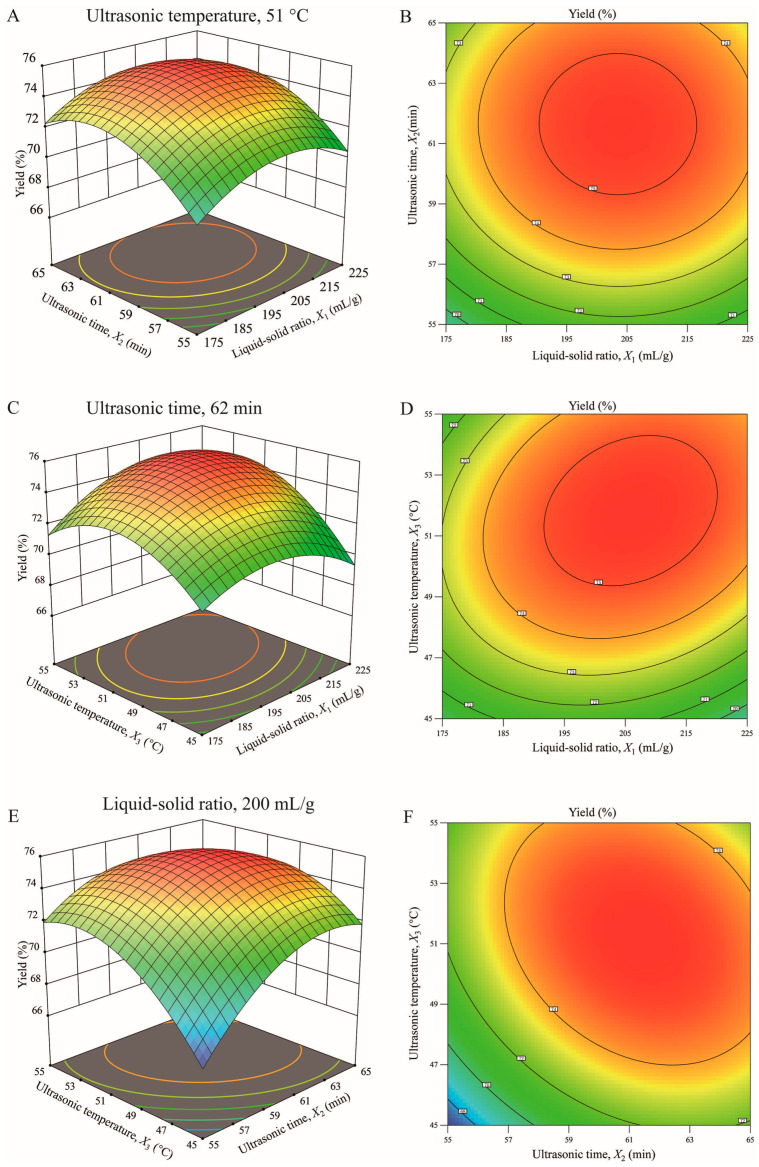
3-D response-surface plots and 2-D contour plots showing effects of various parameters on extraction yield of GJP. (A): The interactive effect of liquid-solid ratio *X*_1_ and ultrasonic time *X*_2_ on yield; (B): The contour plot of yield as a function of liquid-solid ratio *X*_1_ and ultrasonic time *X*_2_; (C): The interactive effect of liquid-solid ratio *X*_1_ and ultrasonic temperature *X*_3_ on yield; (D): The contour plot of yield as a function of liquid-solid ratio *X*_1_ and ultrasonic temperature *X*_3_; (E): The interactive effect of ultrasonic temperature *X*_3_ and ultrasonic time *X*_2_ on yield; (F): The contour plot of yield as a function of ultrasonic temperature *X*_3_ and ultrasonic time *X*_2_. The colors range from light to dark, representing a trend from low to high yield.
